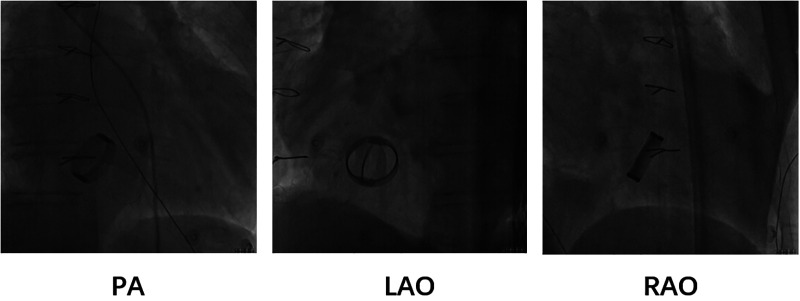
A preoperative chest radiograph of the patient.
